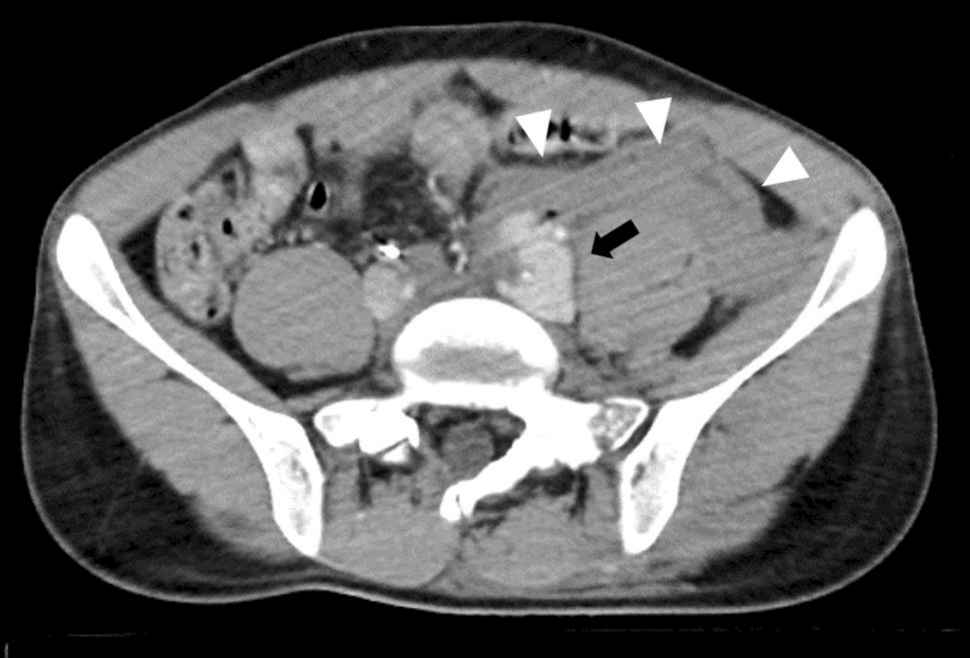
Pelvic contrast-enhancement computed tomography with complete resuscitative endovascular balloon occlusion of the aorta in a patient with multiple trauma showing massive extravasation from the left external iliac vein (black arrow) with a massive hematoma (white arrowheads)
